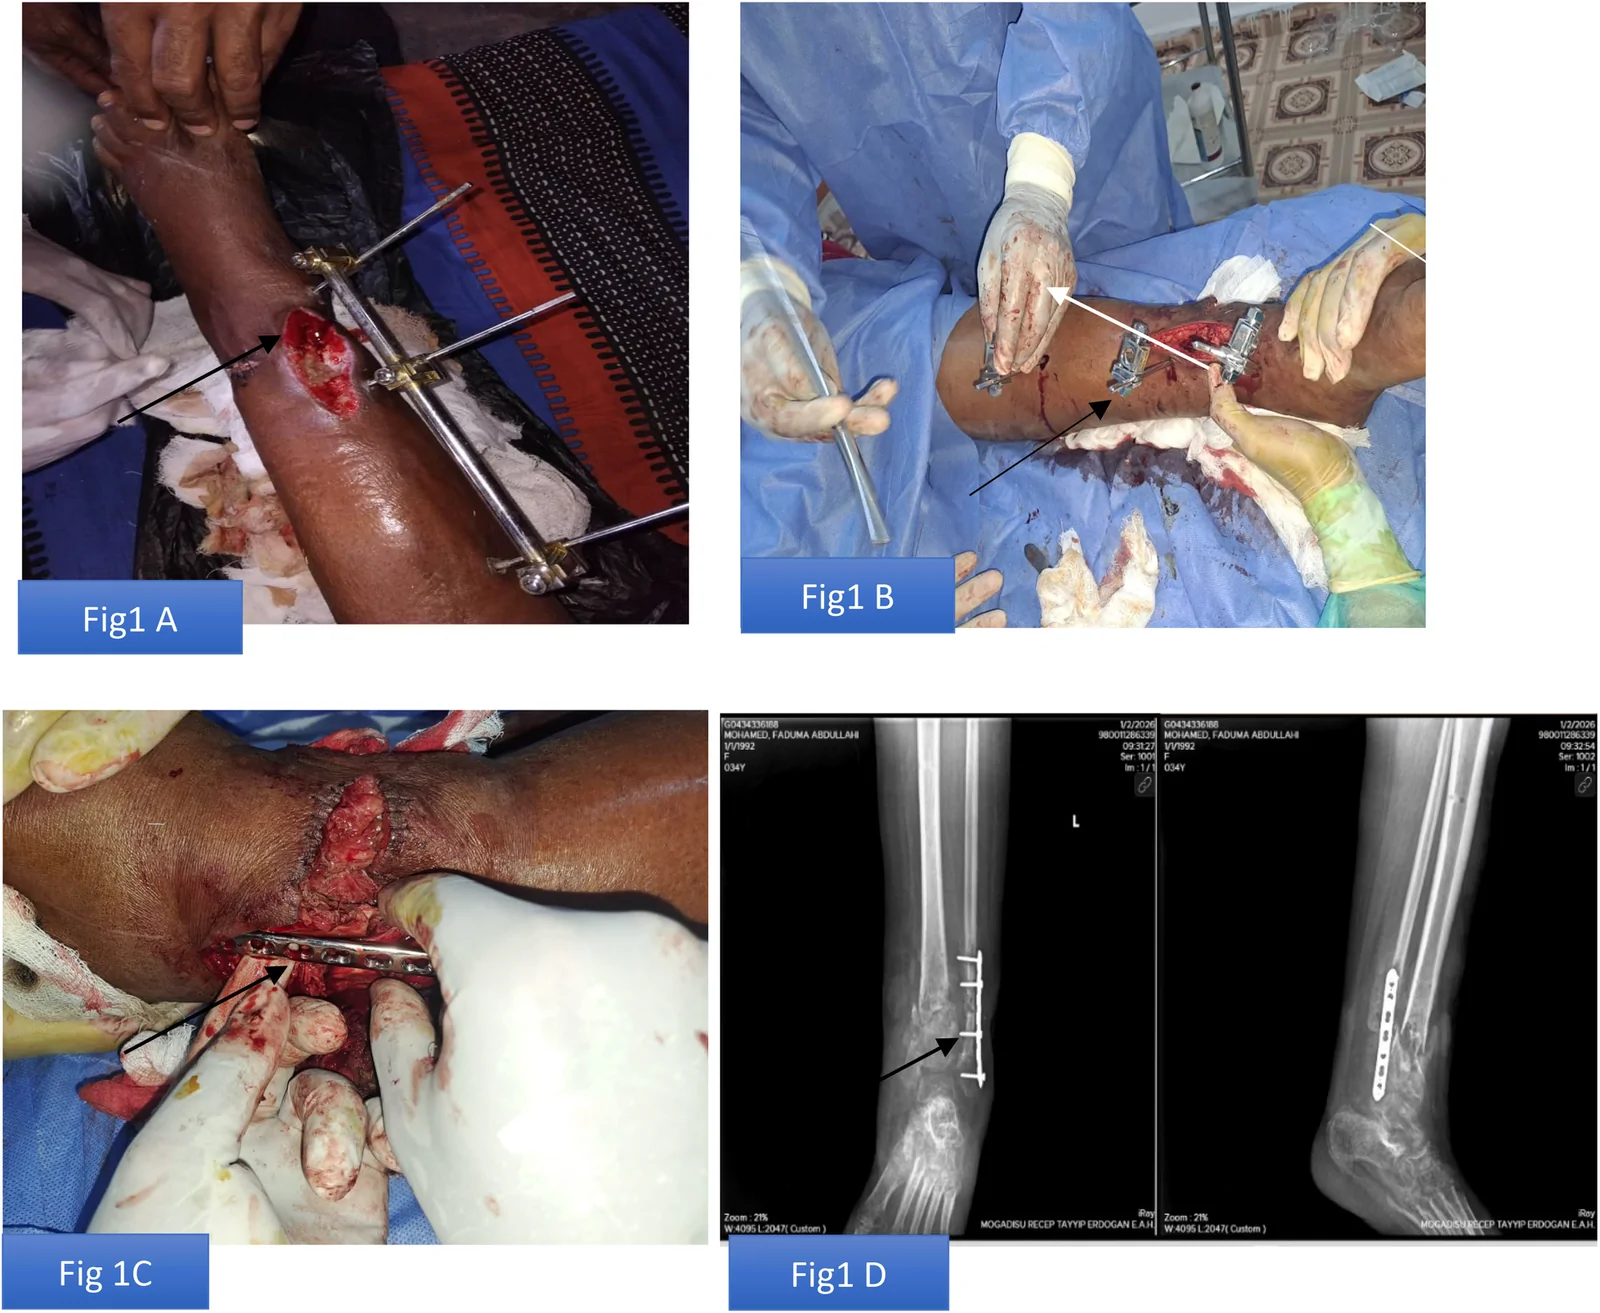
Initial management and early complications following distal tibial fracture fixation of the left leg. (A) Clinical appearance of the left leg after initial external fixation at the referring hospital. The arrow indicates the surgical incision/wound related to treatment and should not be interpreted as an open fracture wound at presentation. The original injury was a closed distal tibial fracture. (B) Clinical appearance during removal of the external fixator at the referring hospital. The arrow indicates the previous external-fixator/pin-site region. (C) Clinical photograph after conversion to internal fixation, demonstrating wound breakdown, exposed fibular fixation, and extensive wound contamination associated with fixation failure. The arrow indicates the affected wound area. (D) Radiograph obtained after conversion to internal fixation, demonstrating fibular plate/screw fixation without direct stabilization of the distal tibial fracture. The arrows indicate the fibular fixation.
